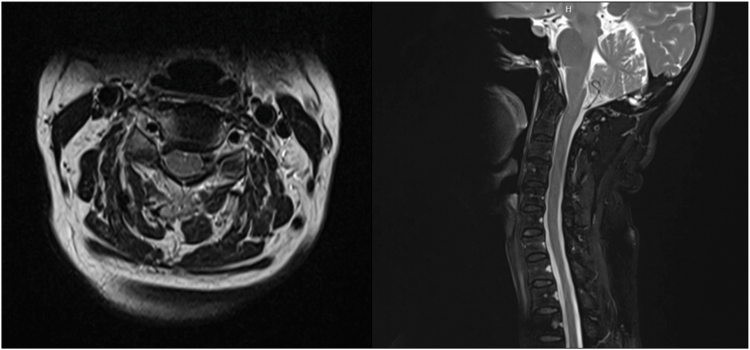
Sagittal and axial MRI views of the cervical spine demonstrating mild spondylosis and canal stenosis without evidence of acute C5-C6 spinal cord or nerve root compression. *MRI*, magnetic resonance imaging.
